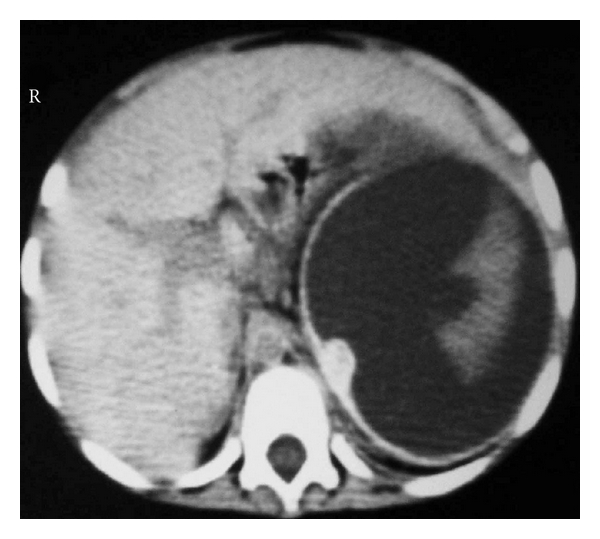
Abdominal CT scan showing a large splenic abscess. Note the sequestrum of splenic tissue in the abscess cavity.
